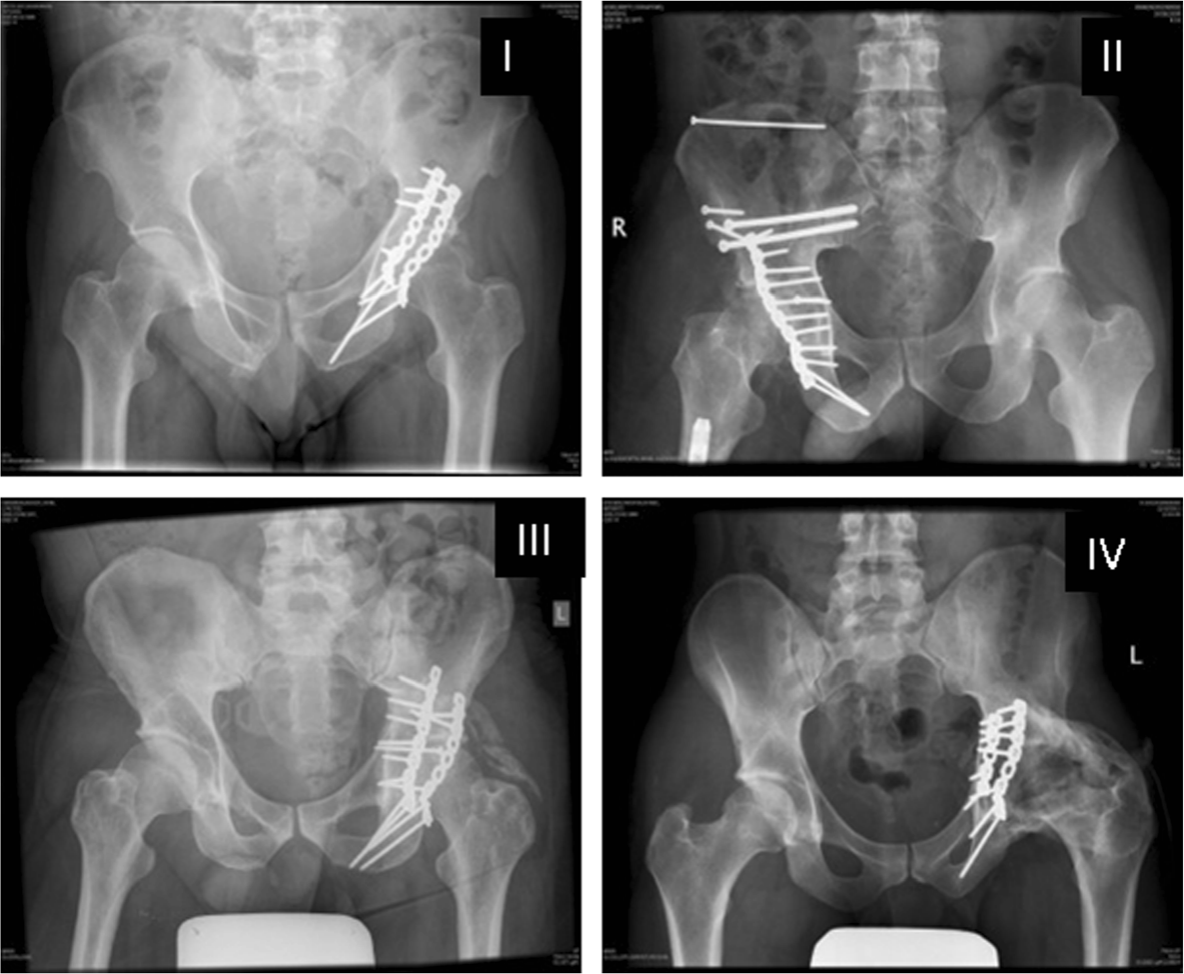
Different grades of heterotopic ossification according to the Brooker classification. I: X-ray of a Brooker class I heterotopic ossification (left hip): ‘islands of bone within soft tissues around the hip’. II: X-ray of a Brooker class II heterotopic ossification (right hip): ‘bone spurs in pelvis or proximal end of femur leaving at least 1 cm between the opposing bone surfaces’. III: X-ray of a Brooker class III heterotopic ossification (left hip): ‘bone spurs that extend from the pelvis or the proximal end of femur, which reduce the space between the opposing bone surfaces to less than 1 cm’. IV: X-ray of a Brooker class IV heterotopic ossification (left hip): ‘radiographic ankylosis of the hip’.
